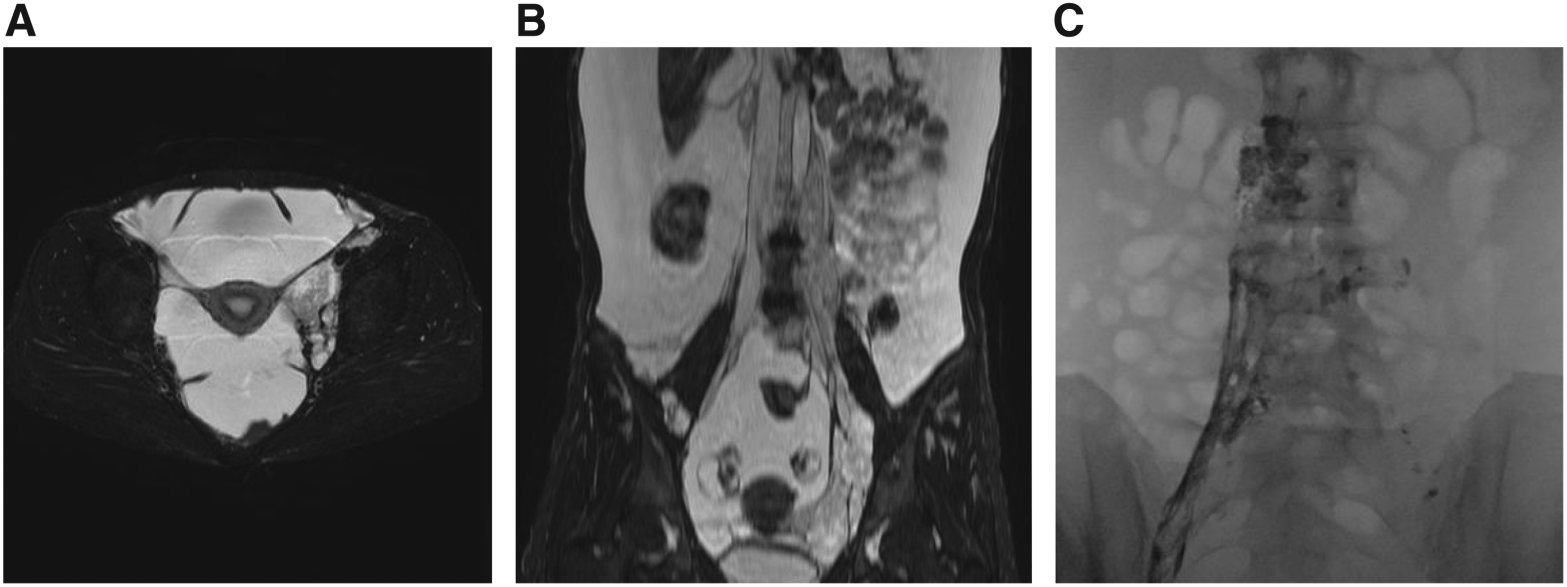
The radiological findings in patients with GLA before sirolimus treatment. (A–C) A 32-year-old woman with GLA showing massive ascites and pelvic LM lesions. (A) An axial view of T2-weighted MRI showing the left LM lesion adjacent to the uterus. (B) An extensive heterogeneous soft mass on the left side extending from the retroperitoneal area to the bladder is shown in a coronal view of T2-weighted MRI. (C) A lymphangiography, spot radiograph of the bilateral groin nodes showing the initial phase of intranodal injection of lipiodol with opacification of the bilateral inguinal lymph nodes. The left side shows inadequate lymphatic flow and leakage of lipiodol to the left abdominal cavity from the lymphatic mass lesion.
